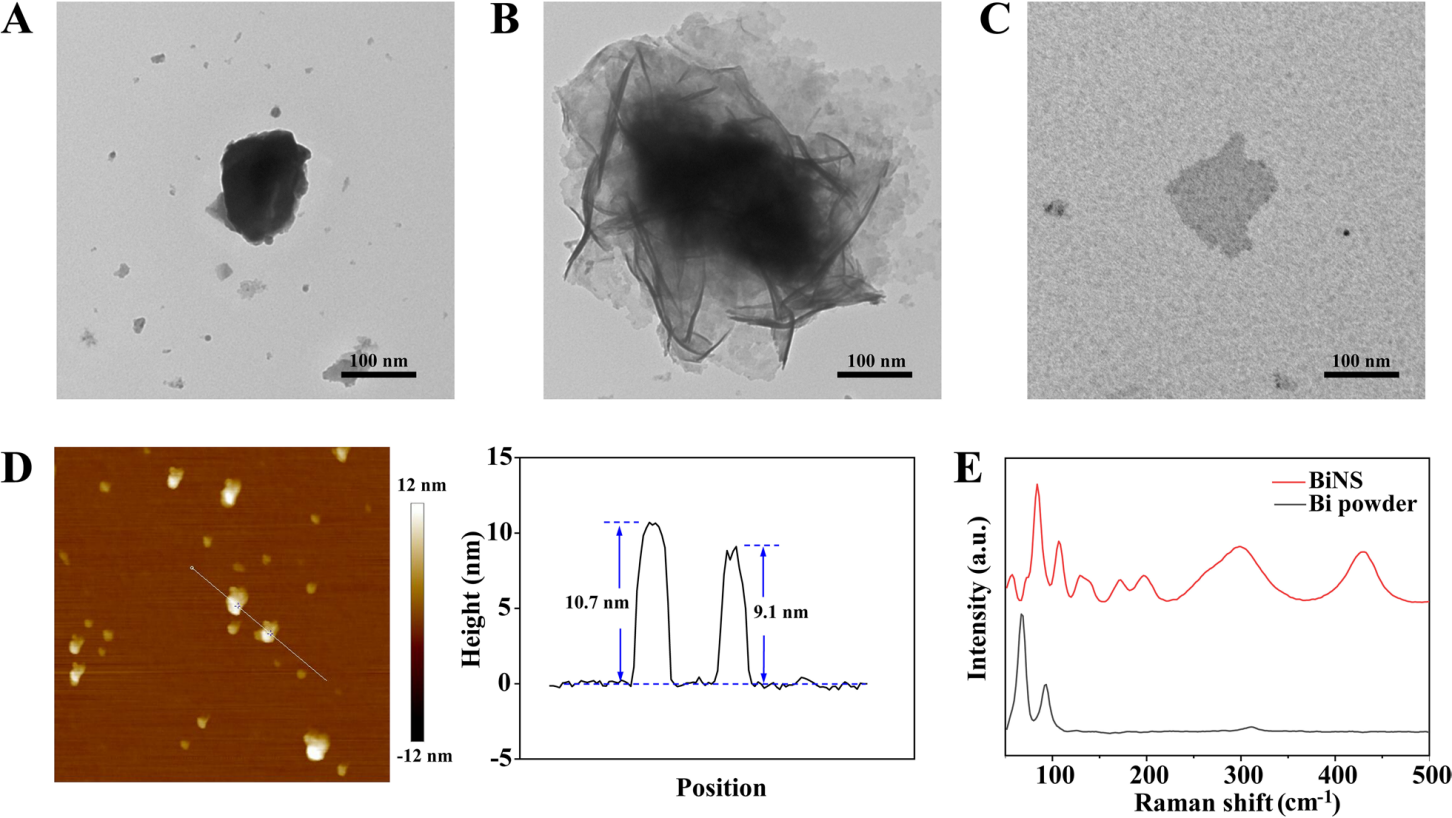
Characterization of BiNS. TEM images of Bi powder with different treatments, including probe ultrasonication for the first time (A), freezing thawing (B) and probe ultrasonication for the second time (C). Scale bar: 100 nm. D AFM image of BiNS and height profiles along the white lines in the image. E Raman spectra of Bi powder and BiNS
